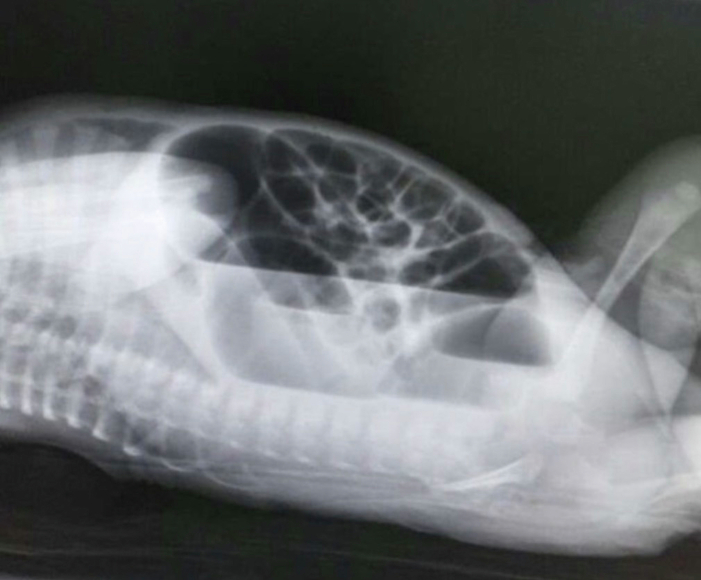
A cross-table abdominal x-ray revealed distended bowel loops with clearly defined air-fluid levels.
